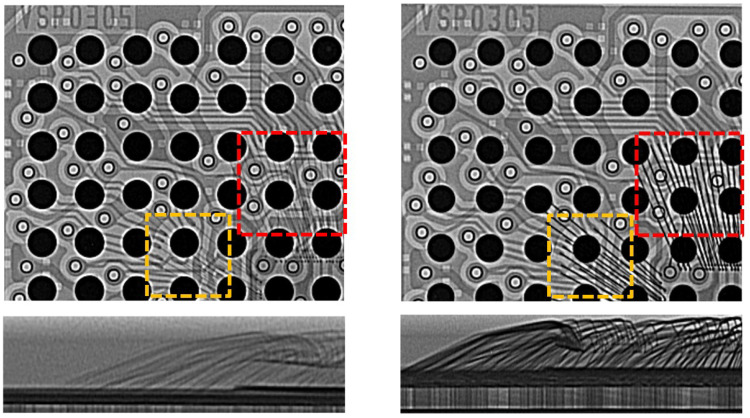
An X-ray image displaying a counterfeit within a Ball Grid Array (BGA) packaging. The genuine gold bonding wire is shown on the right side, and a counterfeit copper one is on the left [60]. The two dashed boxes refer to similar areas within the two samples for comparison.
